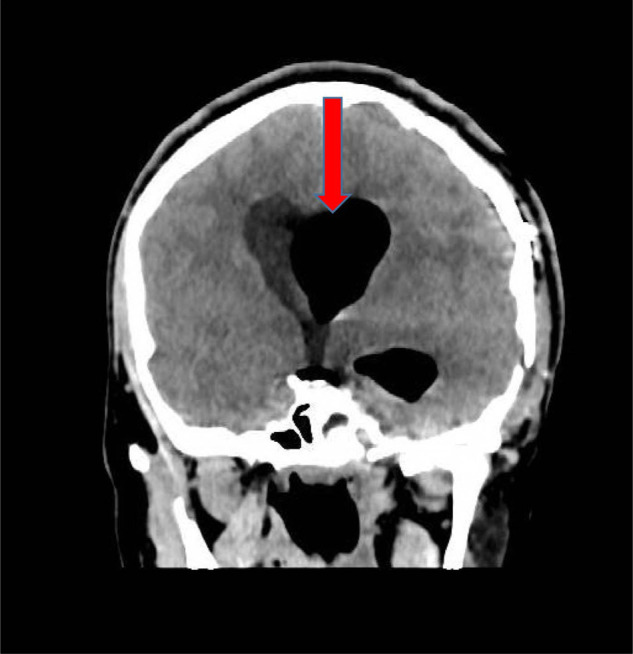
Computed tomography on admission showing tension pneumoventricles (red arrow)
